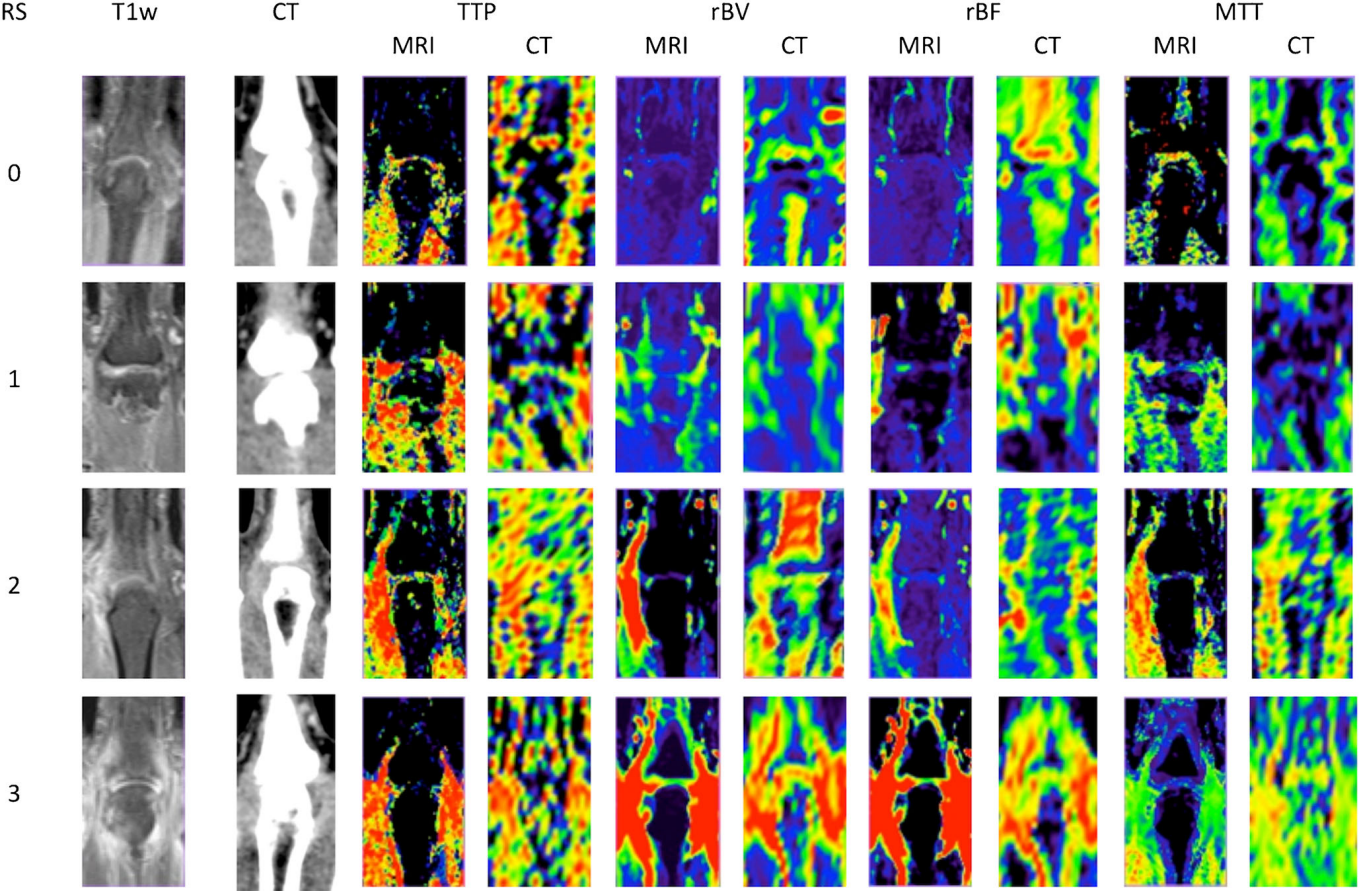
Coronal perfusion maps of metacarpophalangeal joint III for different RAMRIS synovitis scores using standard singular value decomposition in four different patients. RS = RAMRIS synovitis score, MRI = magnetic resonance imaging, CT = computed tomography, TTP = time to peak, rBV = relative blood volume, rBF = relative blood flow, and MTT = mean transit time. The first column shows T1-weighted fat-saturated post-contrast image (T1w) and the second column shows contrast-enhanced CT (CT) images. Perfusion parameters obtained with both modalities increase with RAMRIS scores. For RAMRIS scores 2 and 3, DCE-CT tends to underestimate the severity of synovitis compared to DCE-MRI
